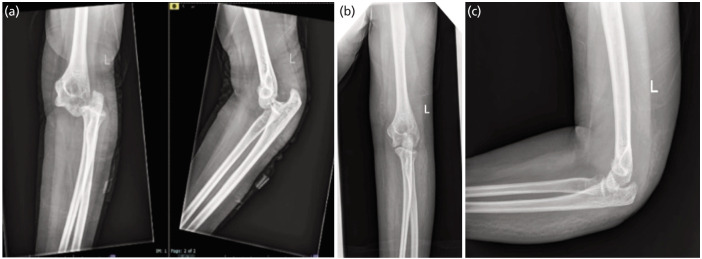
(a) Radiographs of neglected elbow fracture. (b and c) Radiographs after open reduction using posterior approach.
